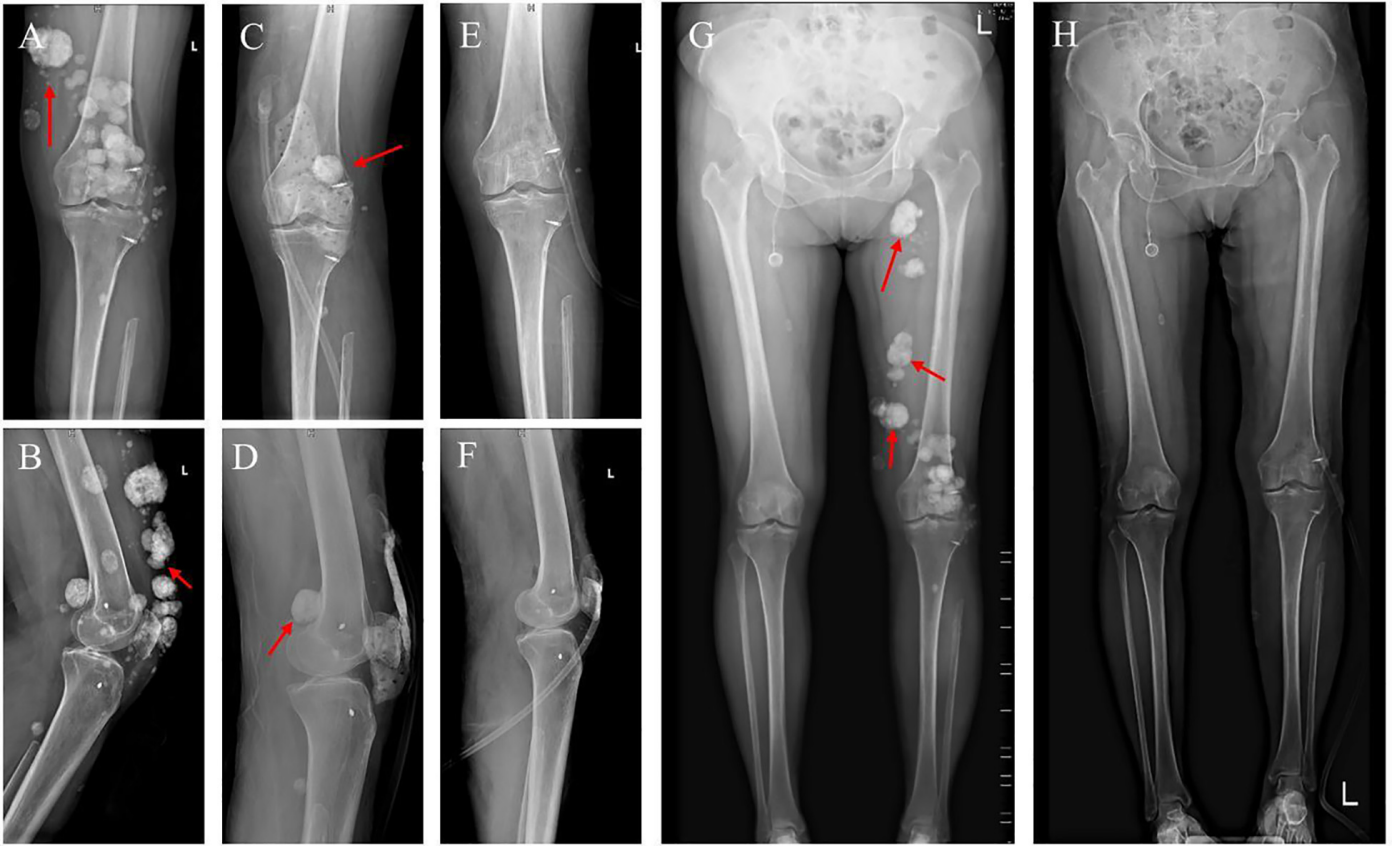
Imaging results before and after surgery. (A, B) Preoperative X-ray of the left knee joint. (C, D) Postoperative X-ray of the left knee joint. (E, F) X-ray after further surgery. (G, H) Full-length standing X-rays of both legs taken before surgery and after the extended resection procedure.
